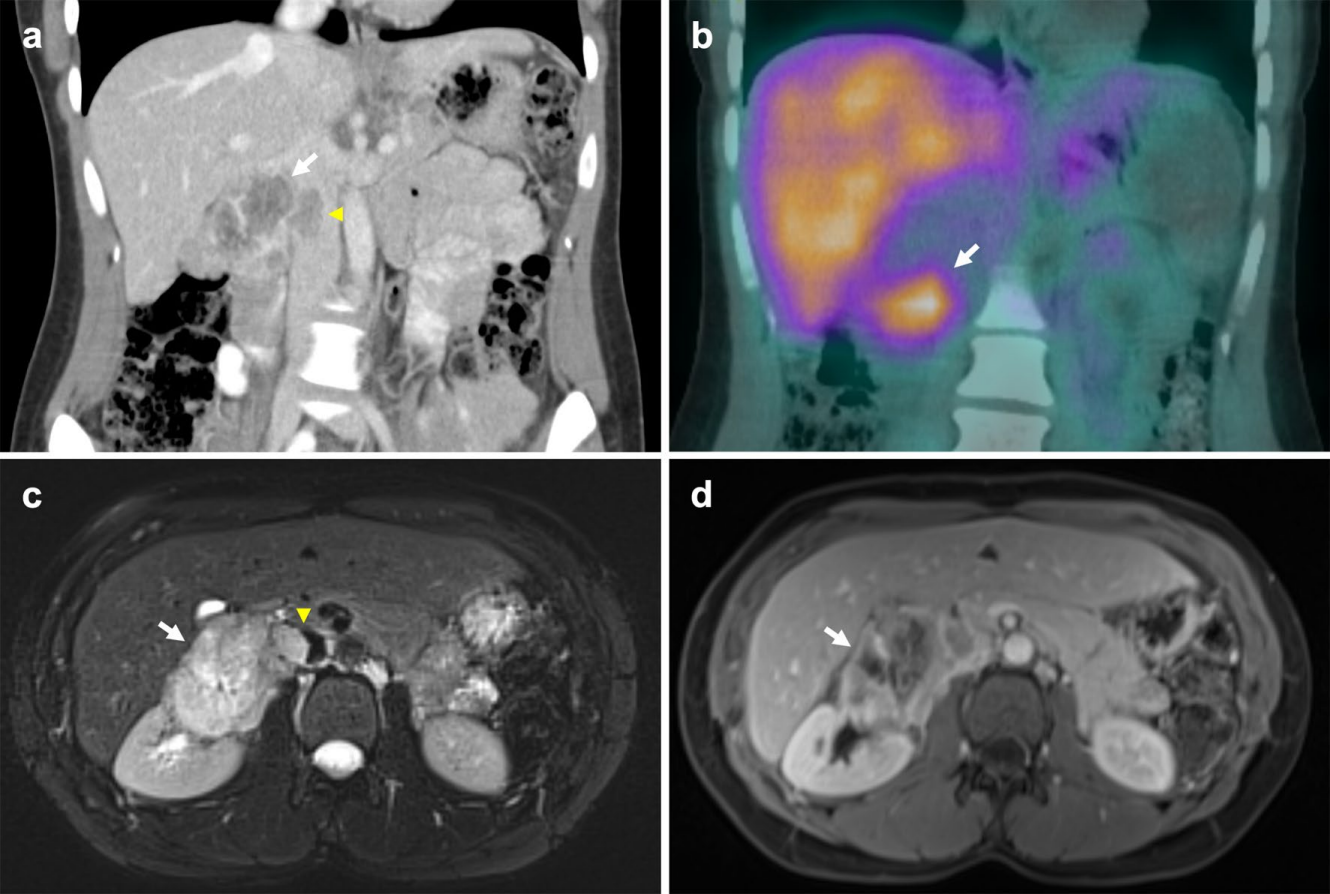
Malignant extra-adrenal phaeochromocytoma invading IVC. a Coronal portal venous phase CT shows a heterogeneously enhancing peri-renal mass (arrow) with possible central necrosis that abuts and extends into the infrarenal IVC (arrowhead). b SPECT CT demonstrates a focus of abnormal MIBG uptake (arrow) within the region of the peri-renal mass. Selected MR images show the retroperitoneal lesion (c) partially obstructing the IVC (arrowhead), demonstrating heterogeneous elevated T2 signal (arrow) on the selected T2-weighted fat-saturated axial image and (d) heterogeneous enhancement (arrow) on the selected T1-weighted fat-saturated axial image
